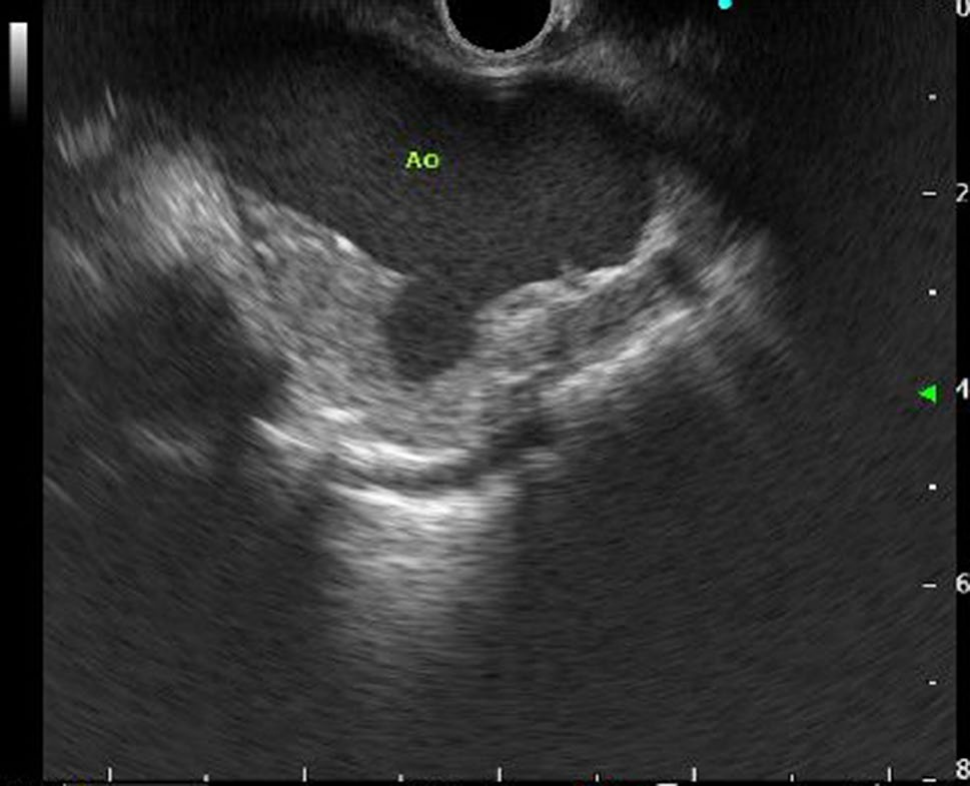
Endoscopic ultrasound from the esophagus revealed aortic wall thickening and a sac-like protrusion of the aortic lumen. *Ao* aorta
